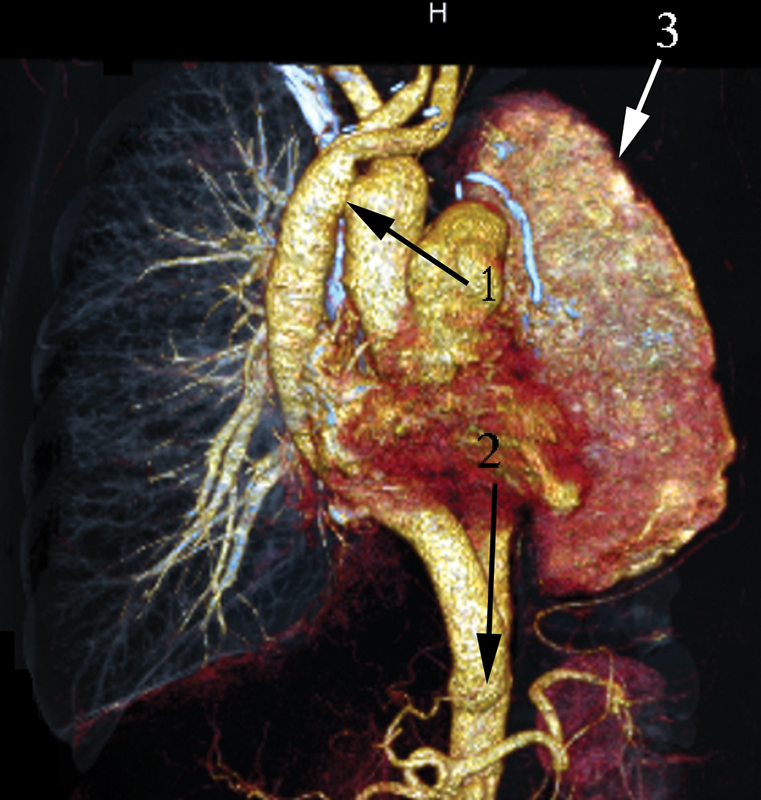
Computed tomography volume rendering of patient 2. Forty months past surgery: 1, the ascending aorta to the graft side-to-side anastomosis; 2, the end-to-side anastomosis of the graft to the abdominal epigastric aorta; 3, the stomach in the vast empty pleural cavity.
